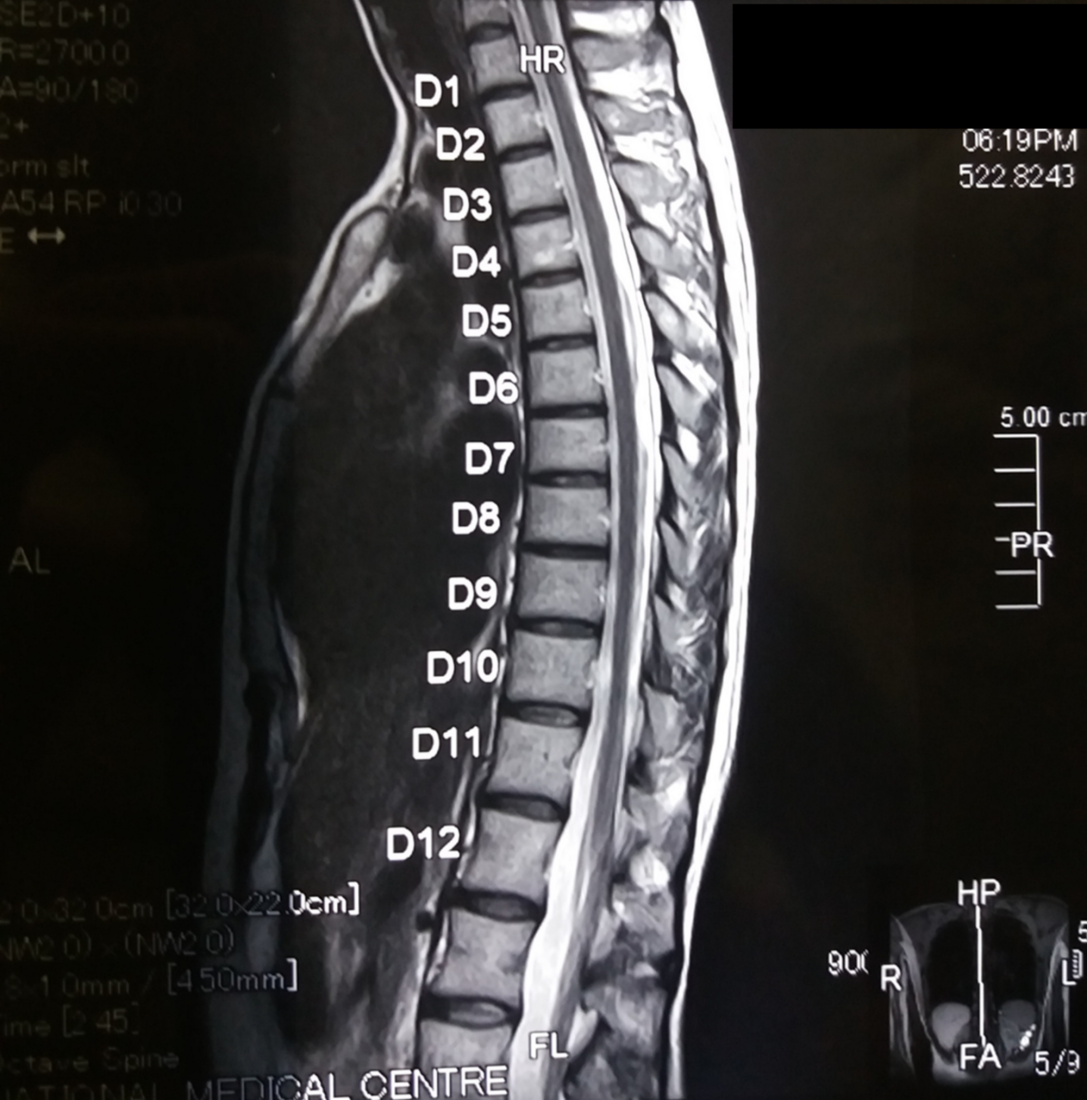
Plain MRI of the thoracolumbar spine did not reveal any pathology MRI: magnetic resonance imaging
